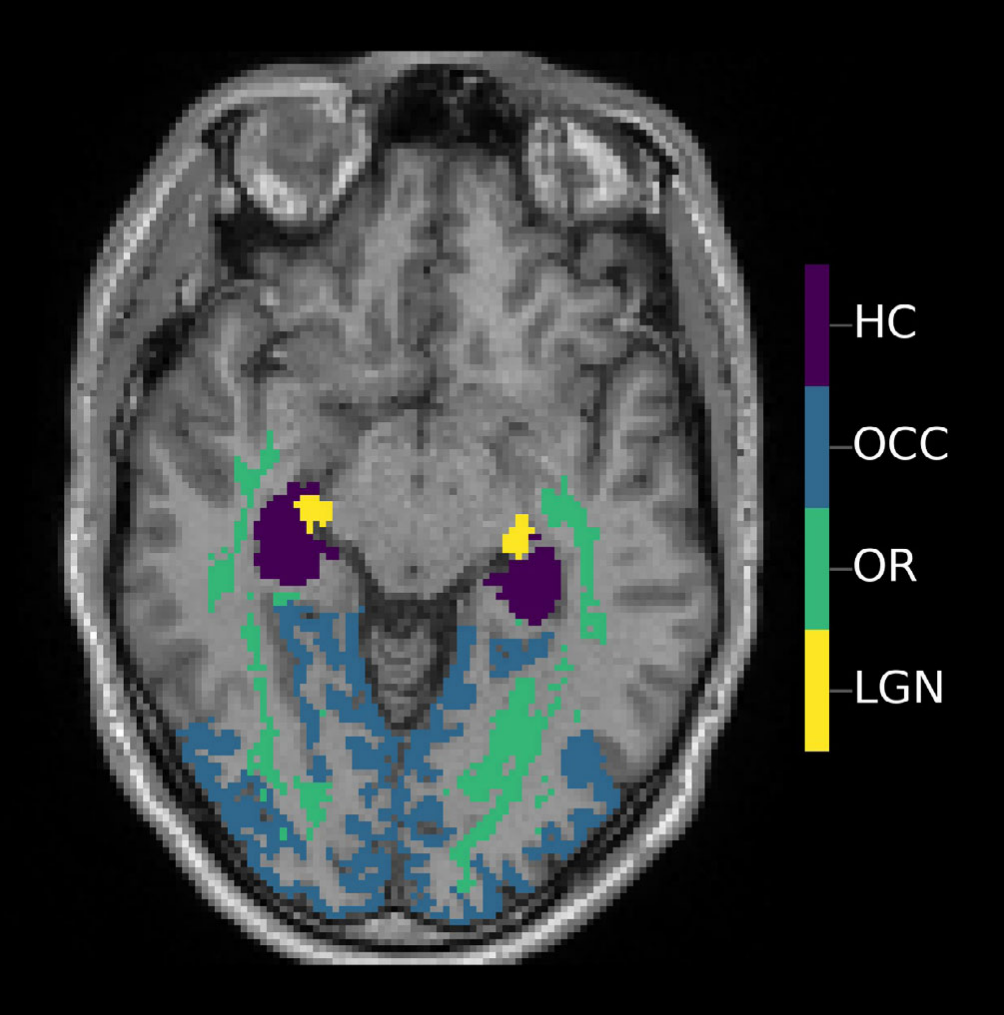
Axial view of brain regions for the lateral geniculate nucleus, optic radiation, pericalcarine cortex, and visual association areas obtained from diffusion magnetic resonance imaging and T1 FreeSurfer processing, and overlaid onto a T1‐weighted scan. HC, hippocampus; LGN, lateral geniculate nucleus; OCC, occipital lobe; OR, optic radiation.
